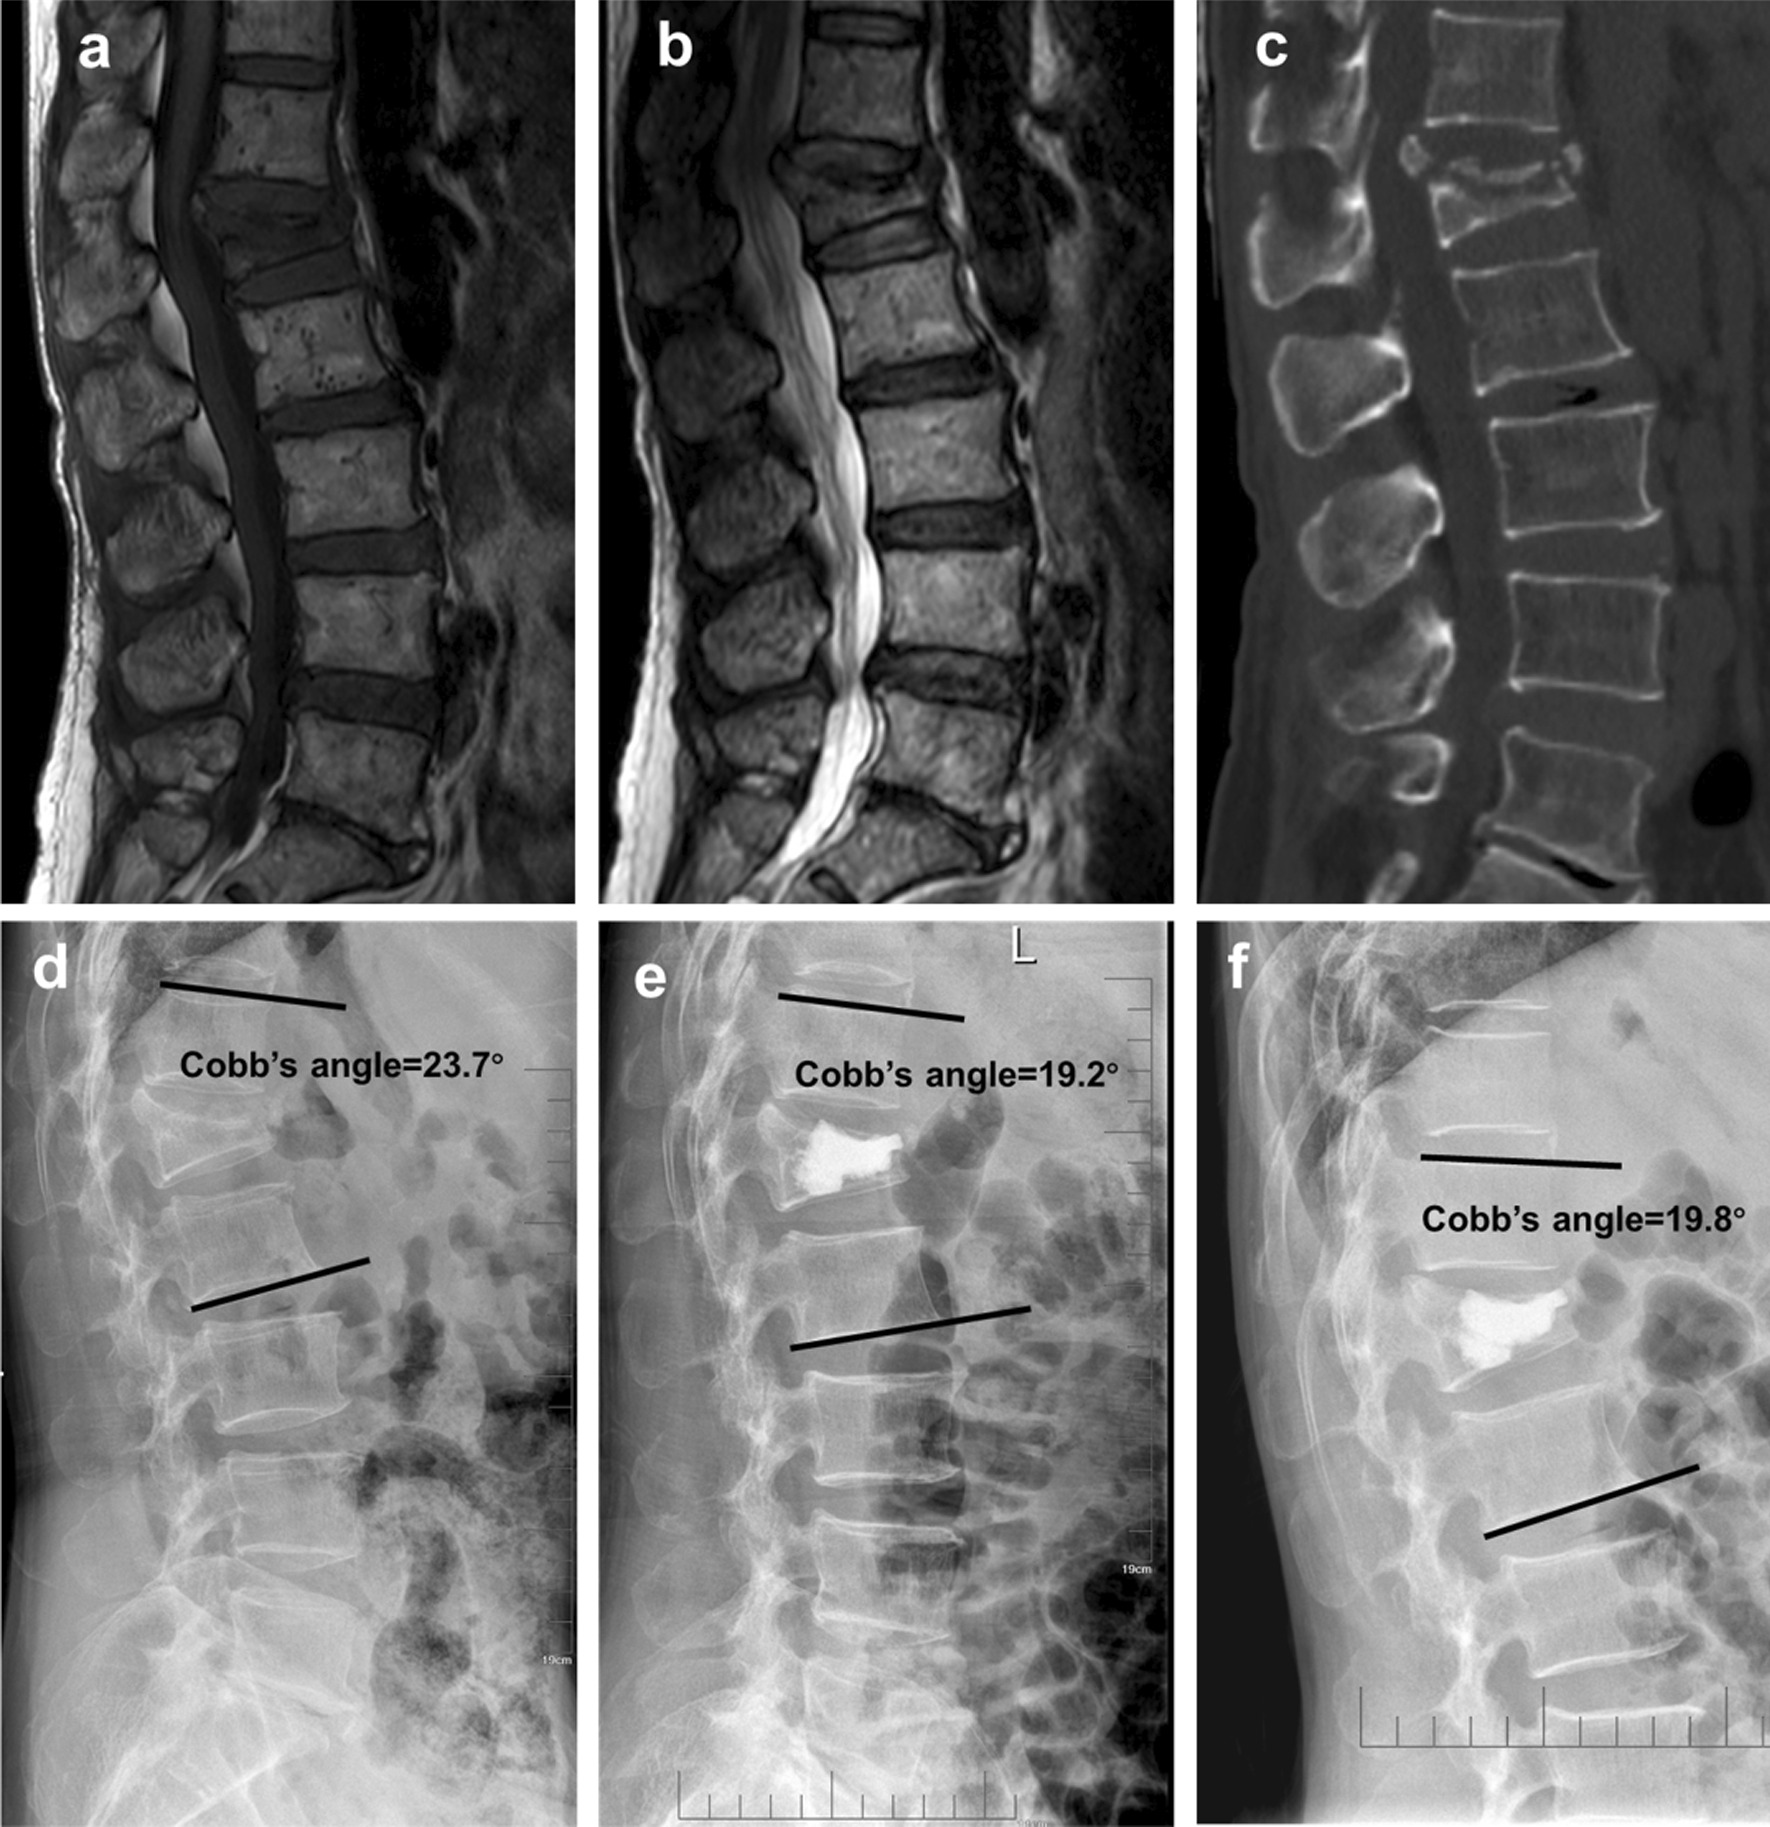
A 63-year-old woman who had L1 stage III Kummell disease without neurological symptom was treated with PVP. a–c The preoperative MRI T1WI, MRI T2WI and CT films showed a chronic osteoporotic vertebral compressive fracture. d–f The preoperative, postoperative and final follow-up X-ray films displayed the vertebral height and Cobb’s angle was recovered a little
